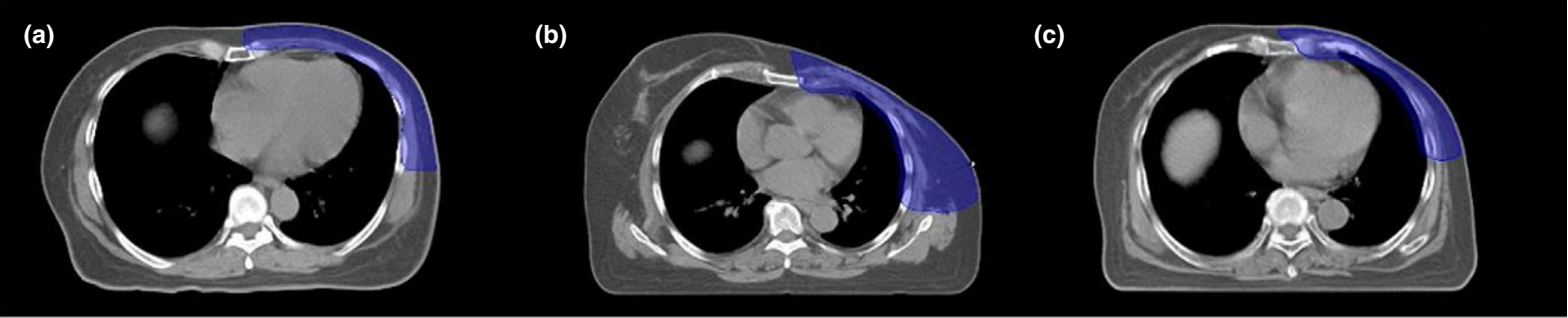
The transverse view of the computed tomography images of planning target volume chest wall structure (blue color) for one patient for each situation (a) the first situation (chest wall only), (b) the second situation (chest wall included supraclavicular lymph nodes), and (c) the third situation (chest wall included supraclavicular and axillary lymph nodes irradiation).
